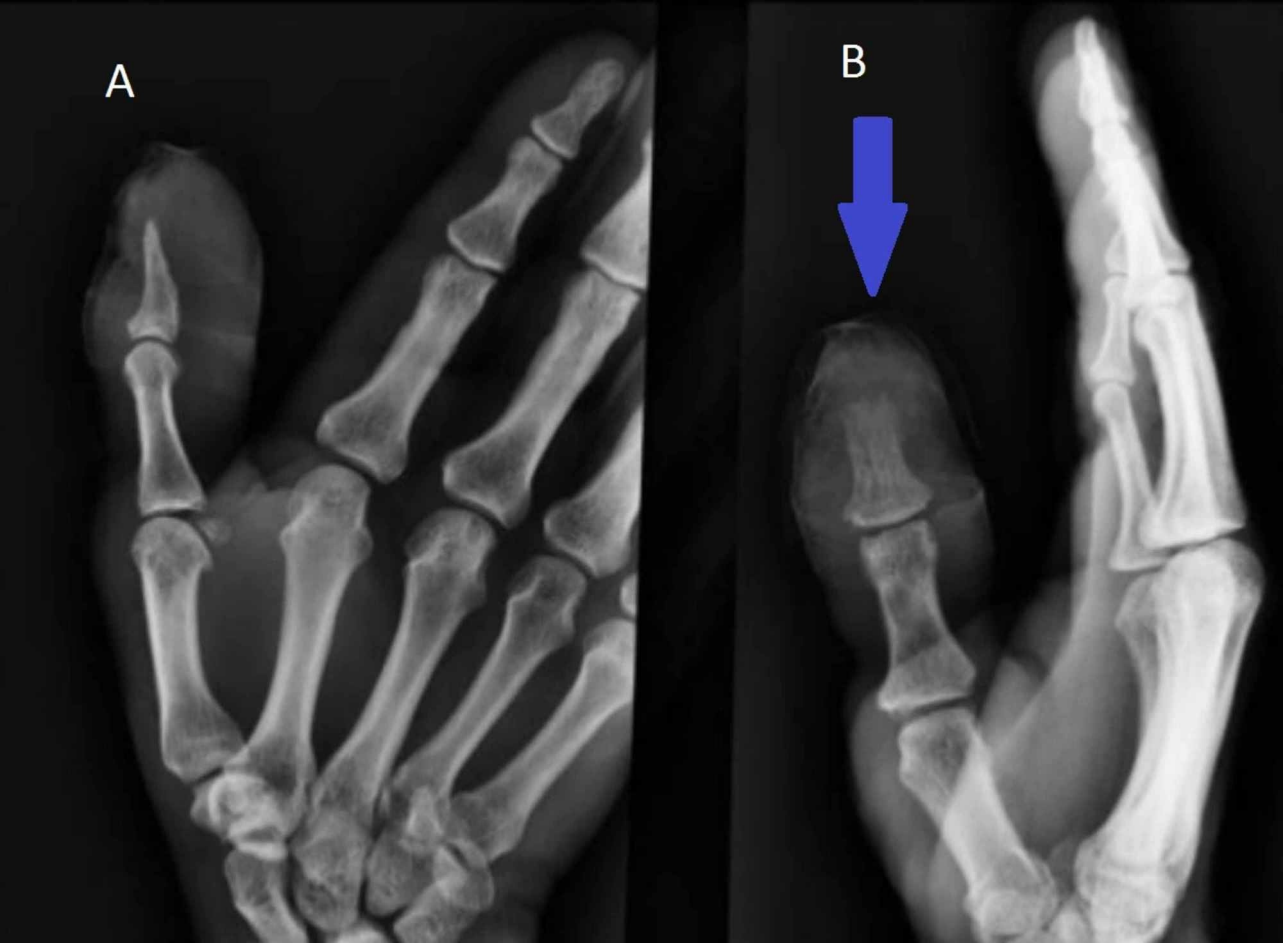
The CT scan of right hand. (A) Antero-posterior view, (B) lateral view. Blue arrow shows soft tissue swelling and osteomyelitis of distal phalanx of the thumb.
